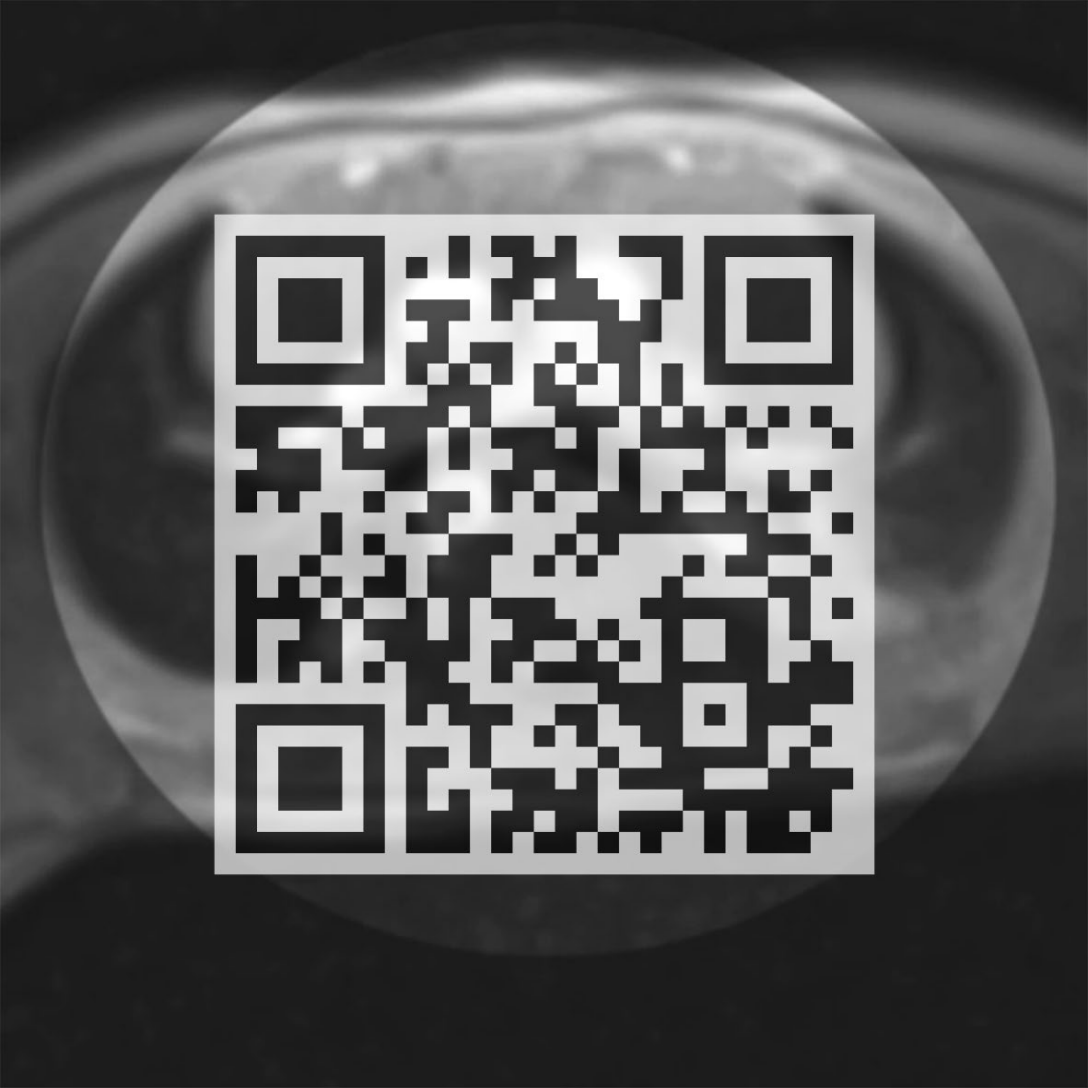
Video 6 A 1.5-year-old boy with stridor. There is a narrow region of the trachea directly behind the pulsating brachiocephalic trunk and straight in front of an aberrant left subclavian artery. Together with the clinical symptoms, this finding is suspicious of an artery compression syndrome. Due to the inflow effect, the vessels display a high signal even in the absence of a contrast medium (proton density weighted MRI)
